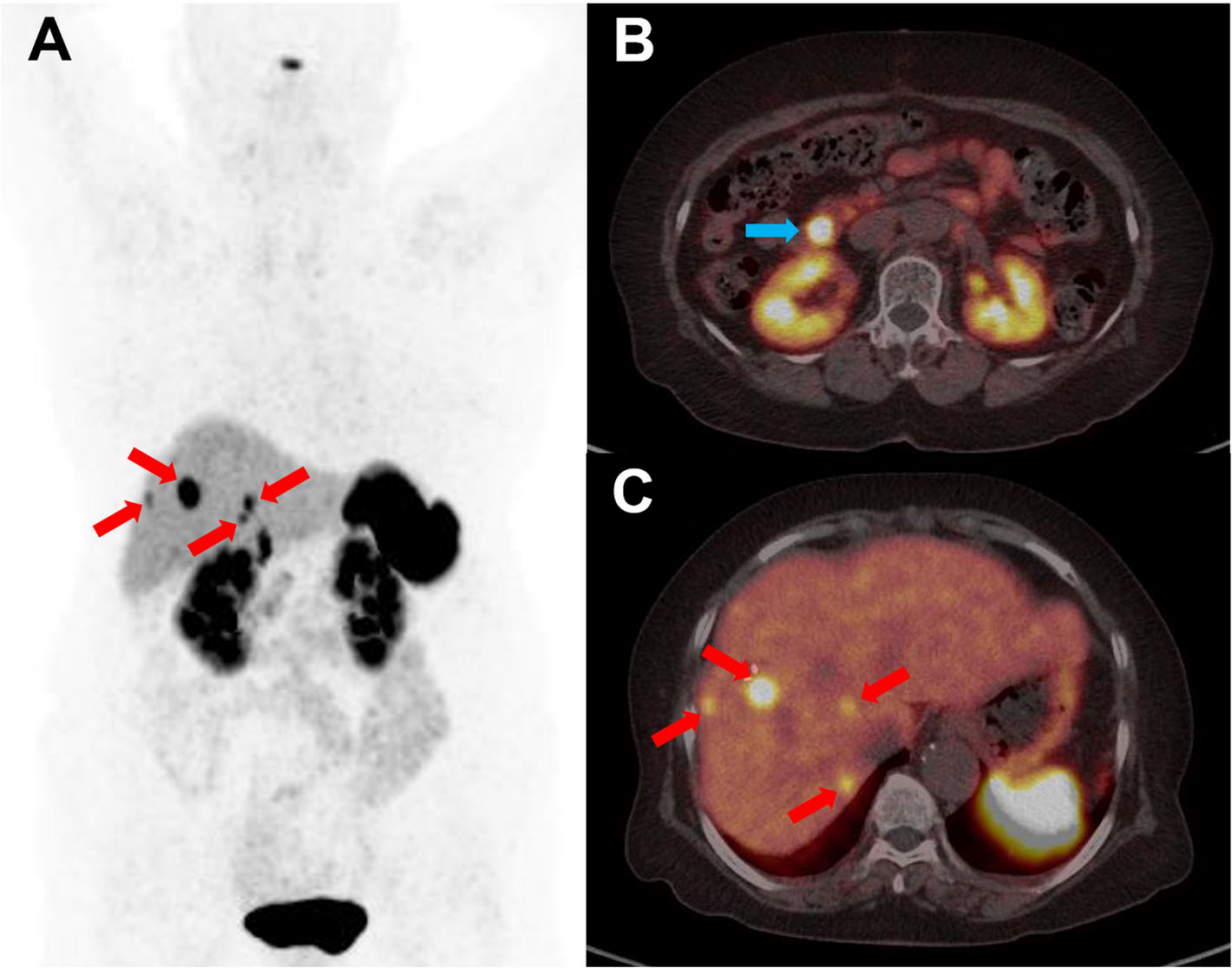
Representative case of a 77-year-old male with a primary duodenal NET grade 2. Maximal intensity projection (MIP, a) and trans-axial (b, c) [^68^Ga]Ga-DOTA-TOC PET/CT images show a primary NET at the duodenal second portion (blue arrow) and multiple small hepatic metastases (red arrows) with intense uptake. He had a good ECOG performance status (0), high TLR (11.8), high SUVmax (53.7), and low WTV (8.5), predicting a good prognosis. After lanreotide therapy, no progression was found until 34 months of follow-up
